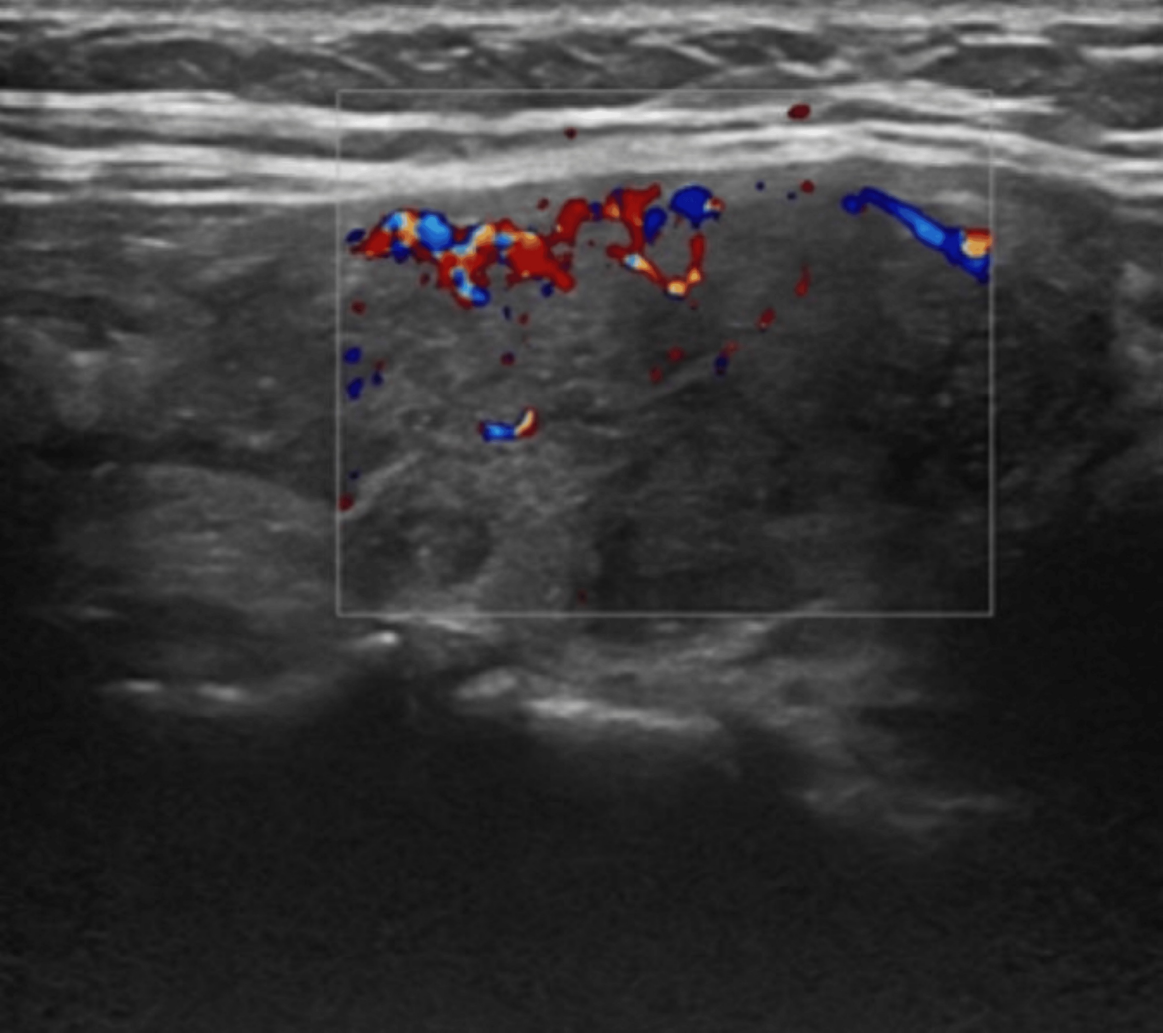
Ultrasound scan of the right lobe of thyroid with overlaid Doppler depicting coarse echotexture and diffuse disease
